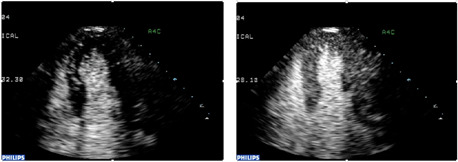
The myocardial contrast echocardiogram obtained in a normal subject. Left panel is taken at the arrival of left ventricular contrast while the right panel shows myocardial opacification delineating myocardial perfusion.
